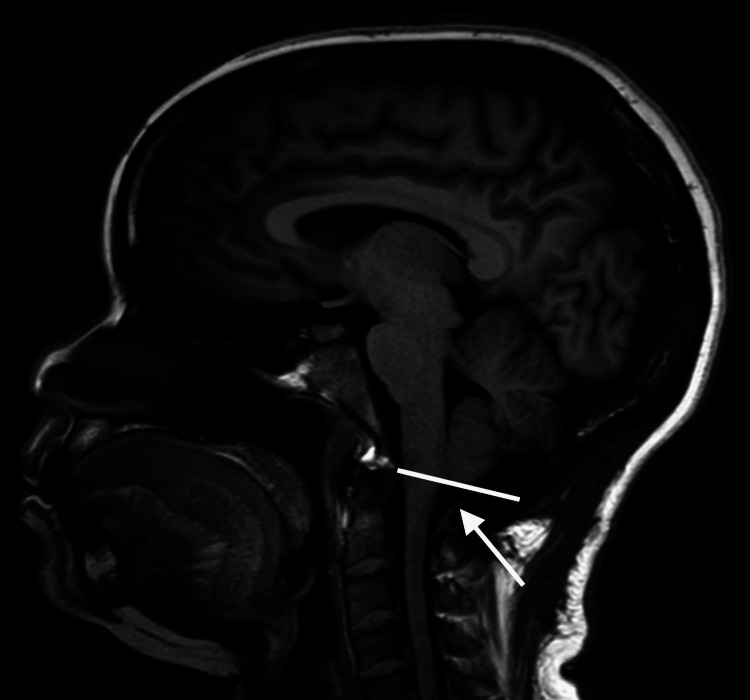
Post-surgical sagittal T1-Weighted MRI brain without tonsillar protrusion
